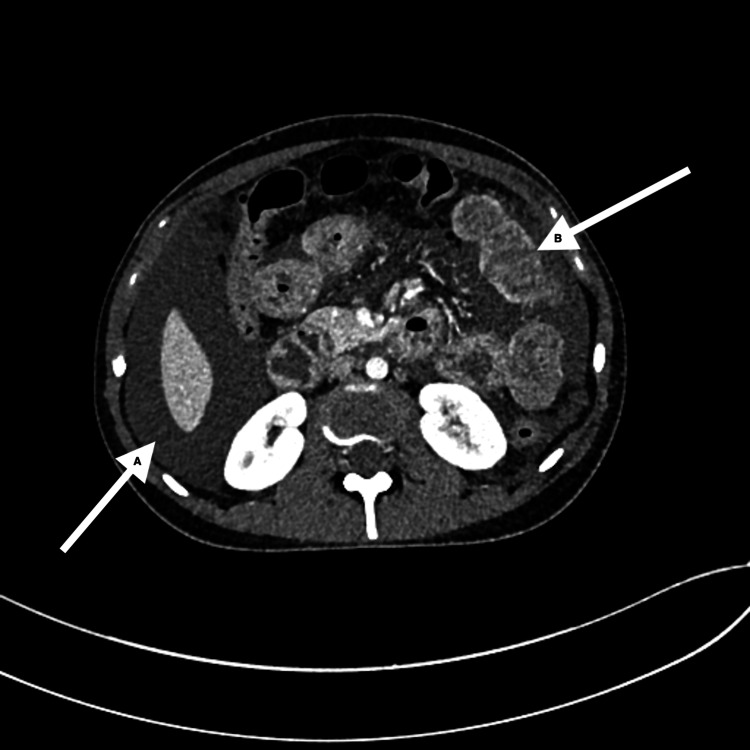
Contrast-enhanced computed tomography (CT) of the abdomen (A) The arrow demonstrates moderate ascites surrounding the liver. (B) The arrow highlights mural thickening and enhancement of small bowel loops.
